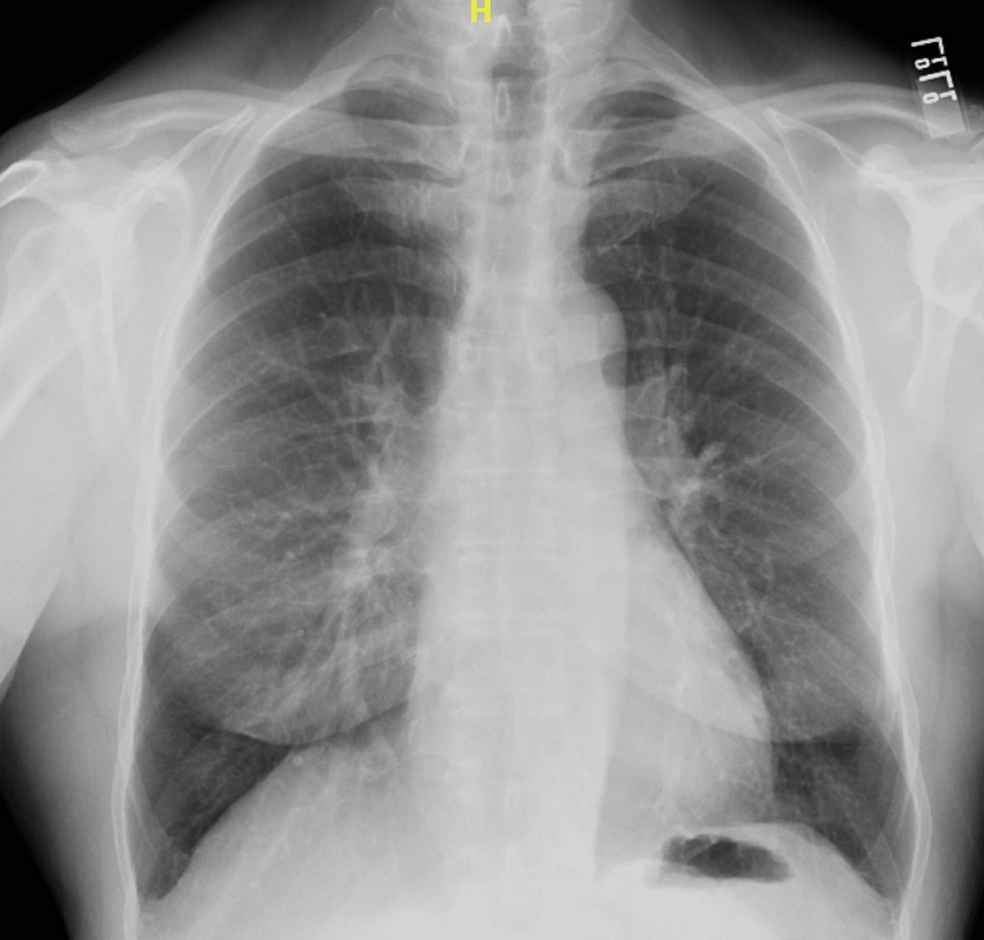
Normal appearing chest X-ray
